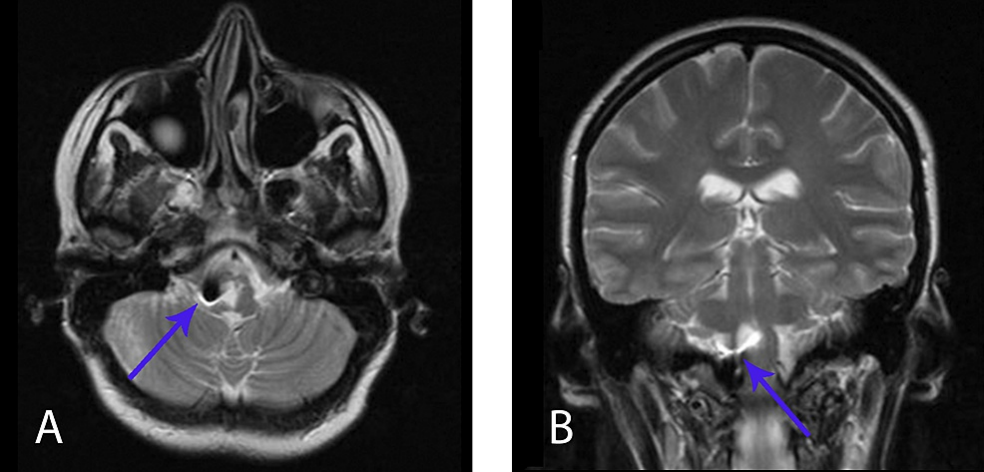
MRI, two months after the second surgery A – MRI Т2 axial view, black point – the mini-clip’s location; B – MRI Т2 frontal view, the stent in the cavity of the cyst
